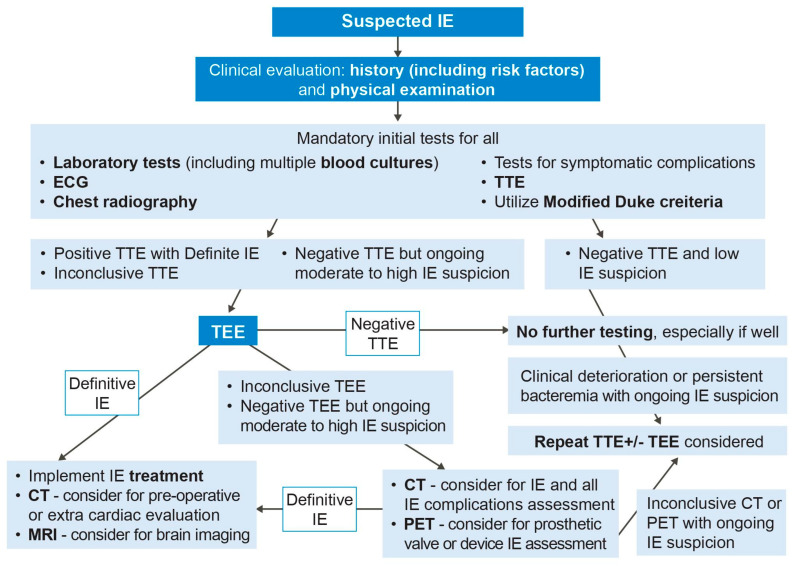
Multi-modality imaging diagnostic algorithm for infective endocarditis. IE = infective endocarditis, TTE = transthoracic echocardiography, TEE = transesophageal echocardiography, CT = computed tomography, MRI = magnetic resonance imaging, PET = positron emission tomography.
